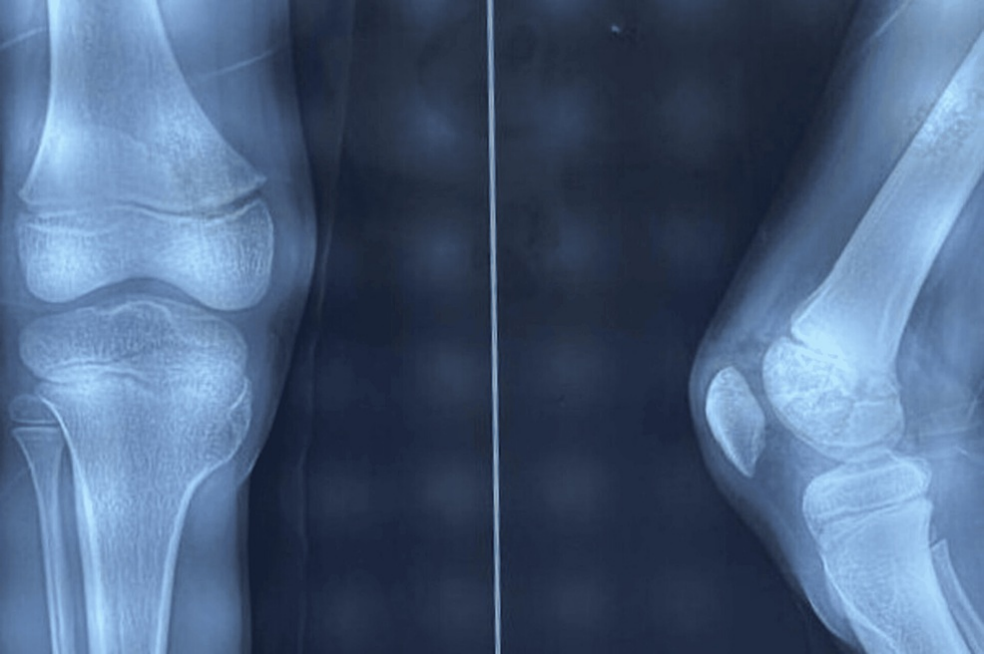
Radiograph at presentation
